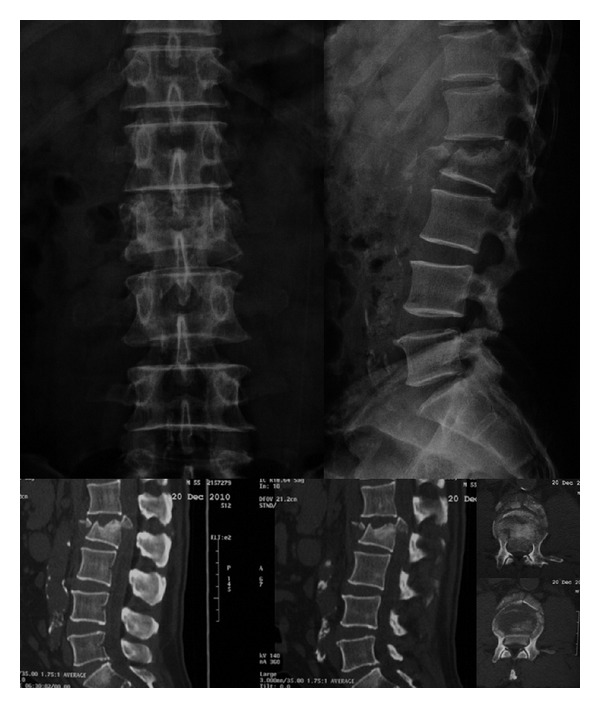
X-ray and CT scan showing a burst fracture on L2 vertebra with a fragment inside the spinal canal.
